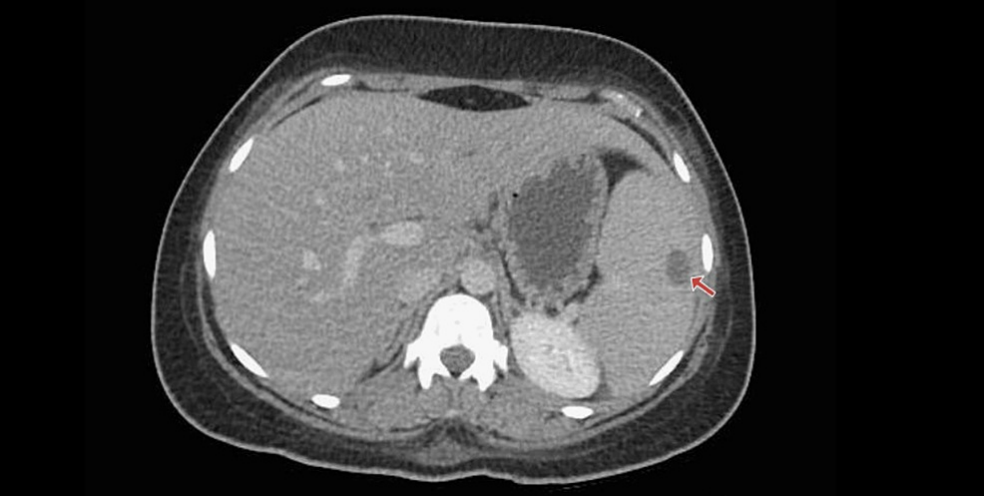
CT of the abdomen shows a hypodense focus in the spleen
